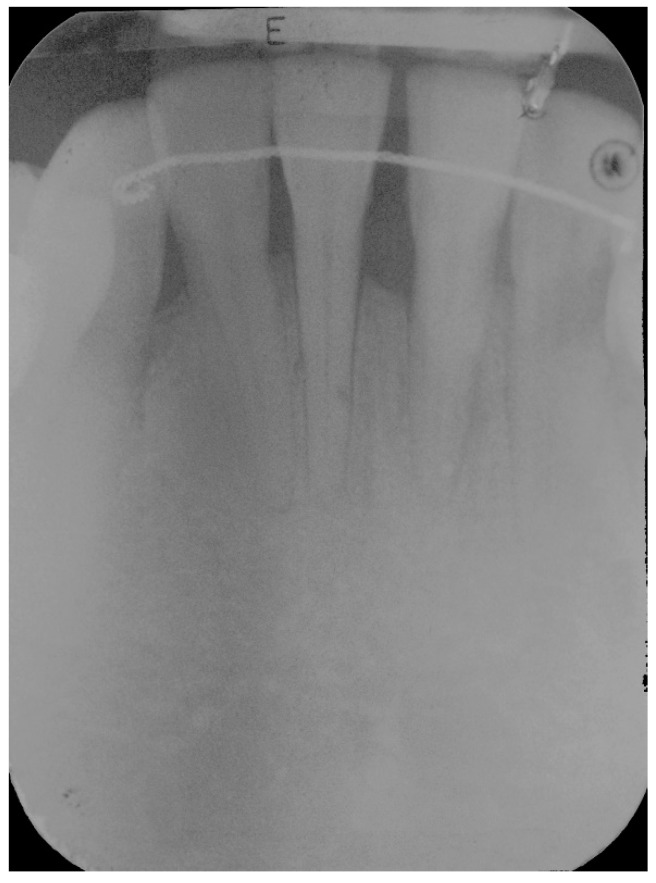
Three years postoperative radiograph, stable results over time can be appreciated.
